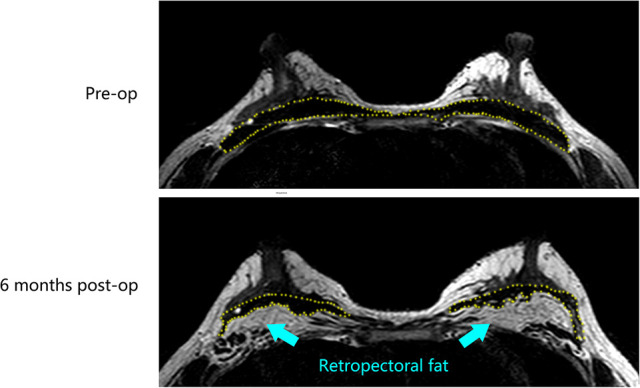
Magnetic resonance images show that a thick fat pad has survived under the pectoral major 6 months after fat grafting. The yellow-dotted line indicates the border of the pectoral major muscle.
